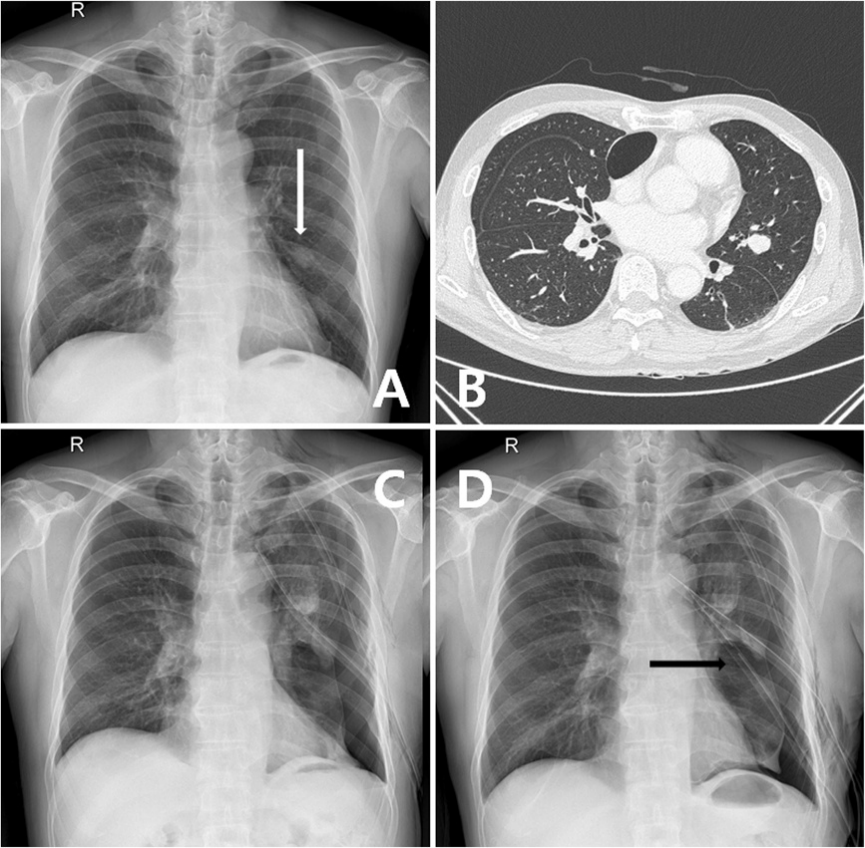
Peri-operative X-ray findings. Chest X-ray (A) and computed tomography (B) show a metastatic lung cancer. A post-operative persistent air leakage results in collapse of the left lungs (C). While we added a chest tube, the lungs could not be fully expanded (D).
